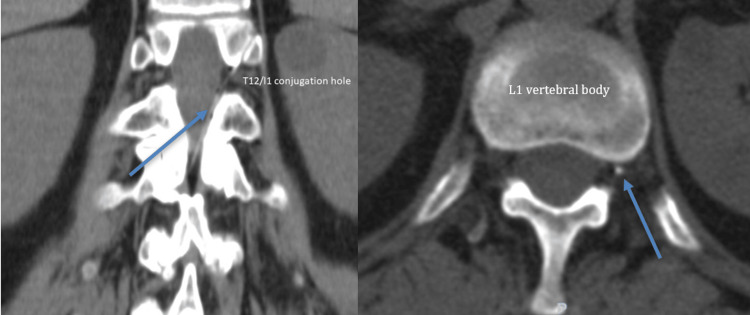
Coronal and transverse CT scan after administration of radiopaque contrast through the epidural catheter in case 2 The left arrow shows the catheter pathway inside the epidural space with ascending and left anterior trajectory and exiting through the T12/L1 conjugation hole. The right arrow shows the approximate final position of the catheter tip at L1 level left paravertebral space.
